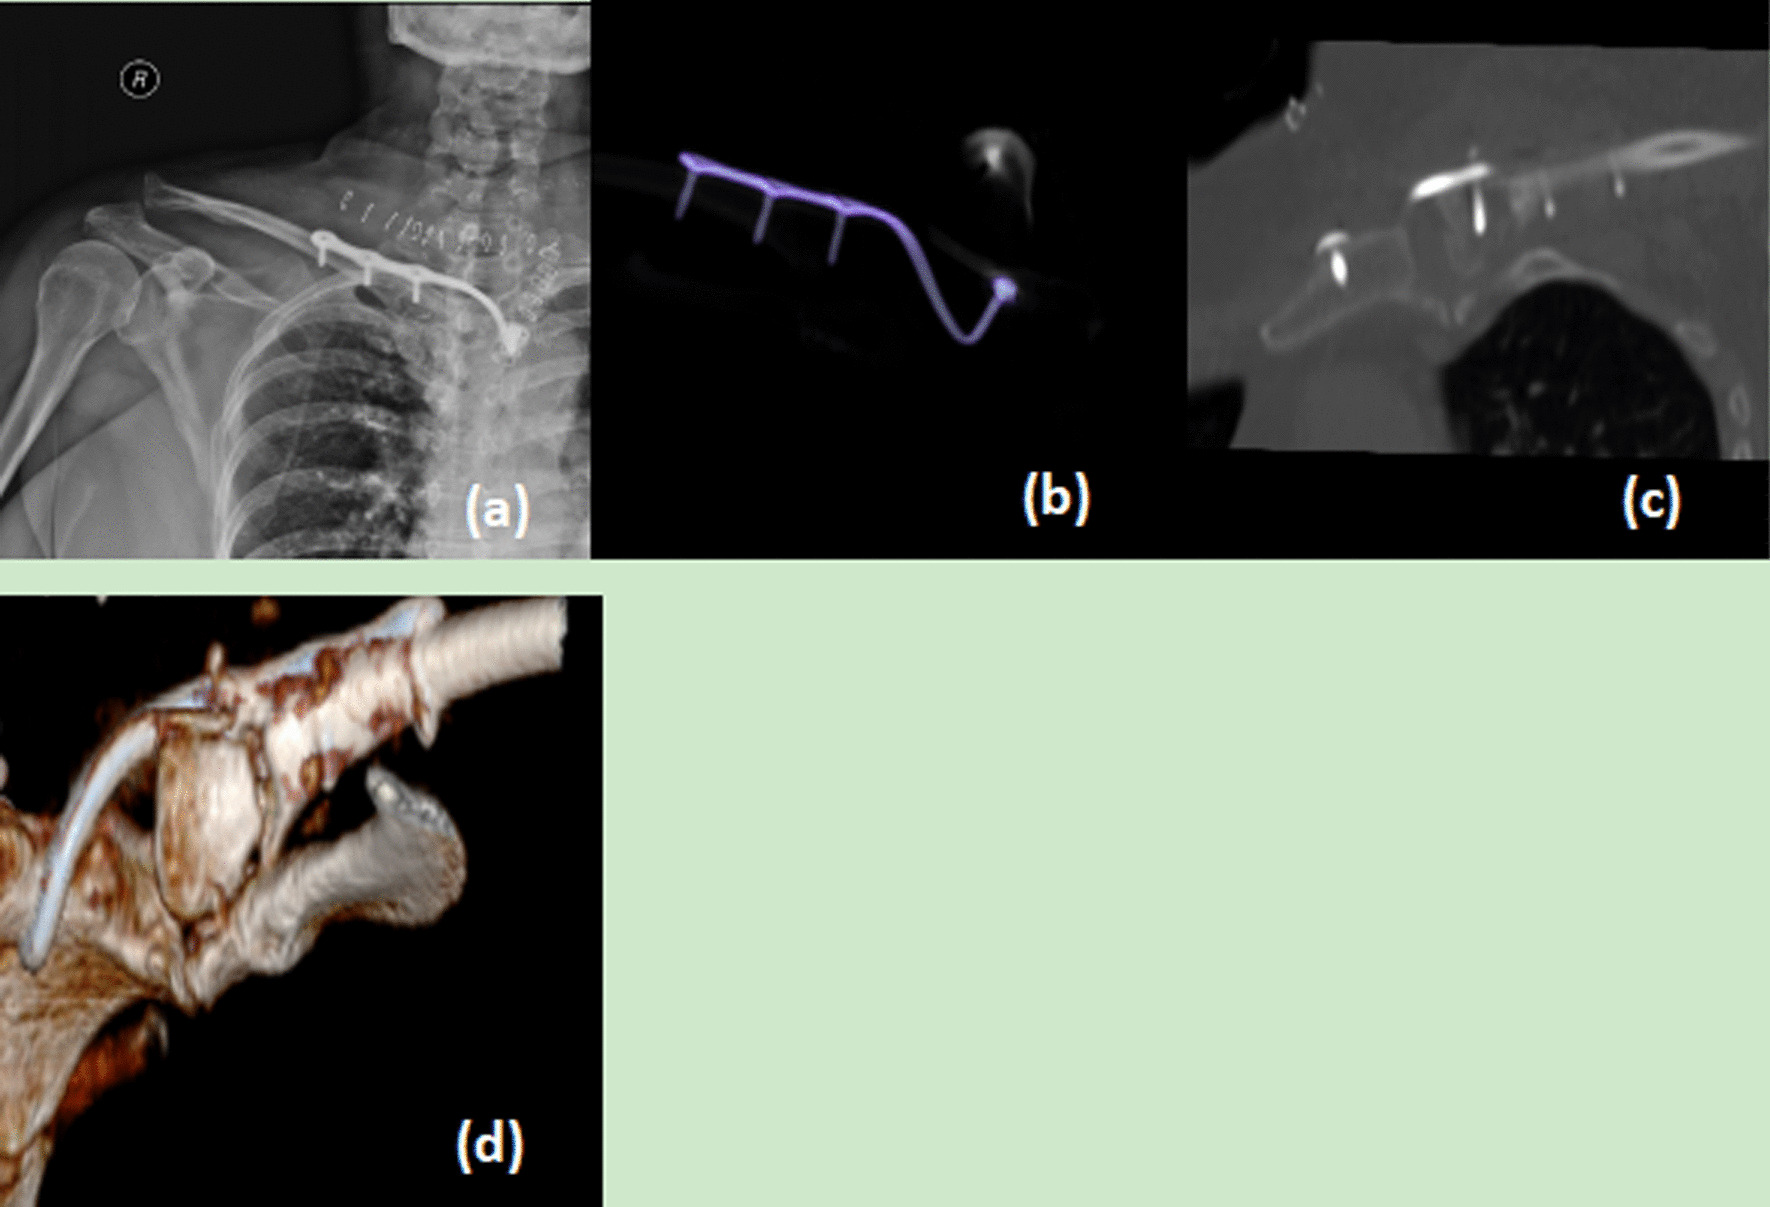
a, b The postoperative X-ray image showed the well reduction by CanHSH sternoclavicular hook plate, c, d postoperative CT scan and three-dimensional CT scan of the patient after 3 months follow up
